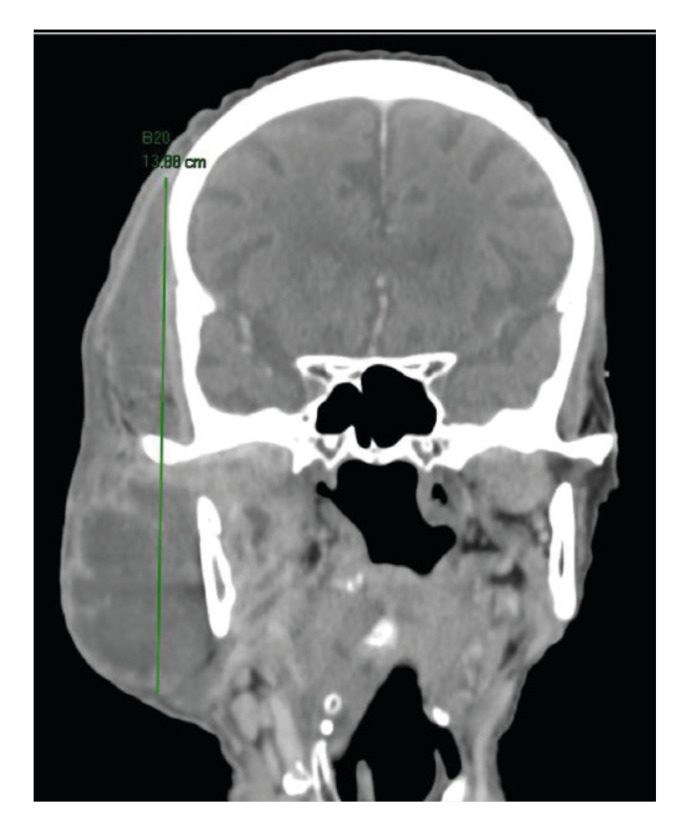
Contrast-enhanced computed tomography showing the presence of a deep neck abscess involving the buccal, masticator, parotid, and temporal spaces.
